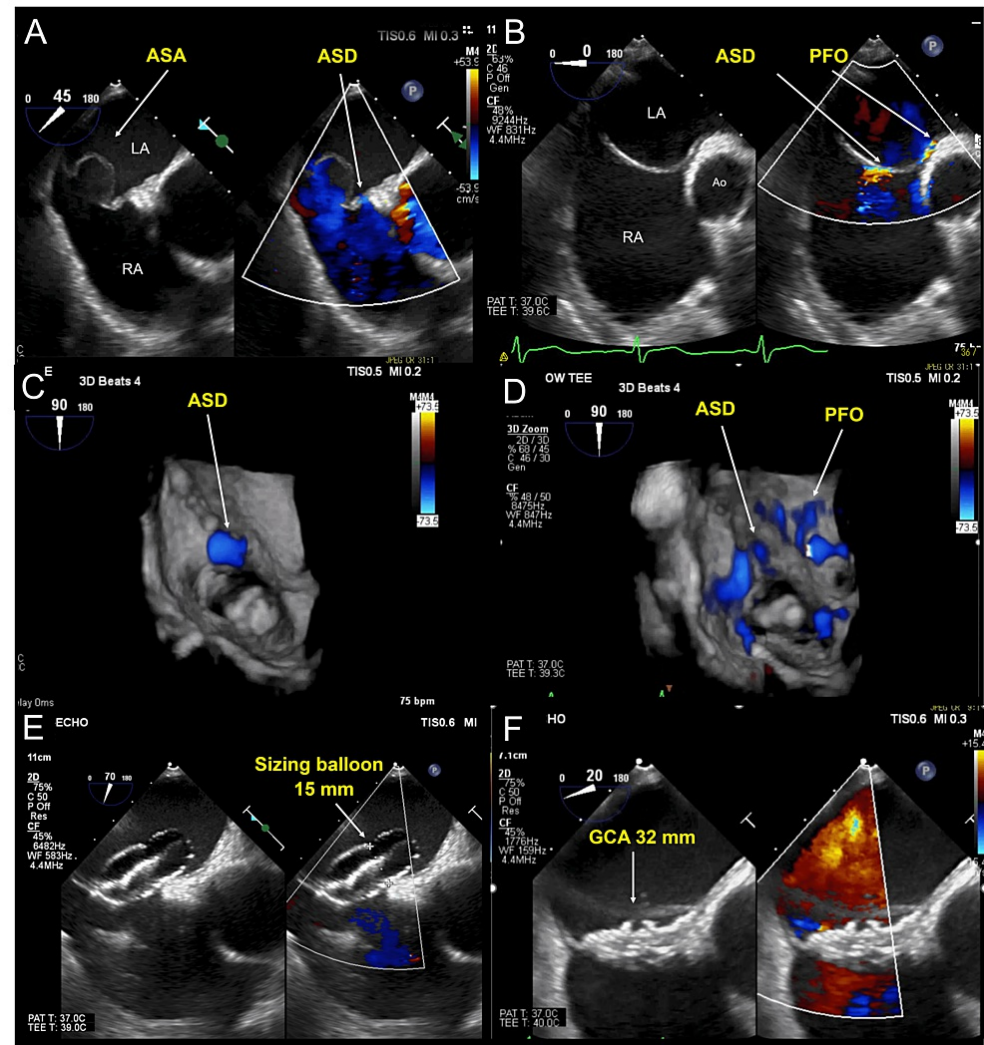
TEE imaging for measurement in ASD, PFO, and ASA and deployment of a 32-mm GCA device (A) TEE showed a partial anterior rim defect and floppy ASA in the posterior rim in both directions of the left and right atrium. (B) A PFO was also present. (C and D) ASD and PFO by 3D TEE. (E) The sizing balloon at stop-flow through the ASD was 15 mm. (F) A 32-mm GCA was implanted based on the sizing balloon calculation. 3D, three-dimensional; ASA, atrial septal aneurysm; ASD, atrial septal defect; GCA, GORE® Cardioform ASD Occluder; PFO, patent foramen ovale; TEE, transesophageal echocardiography
